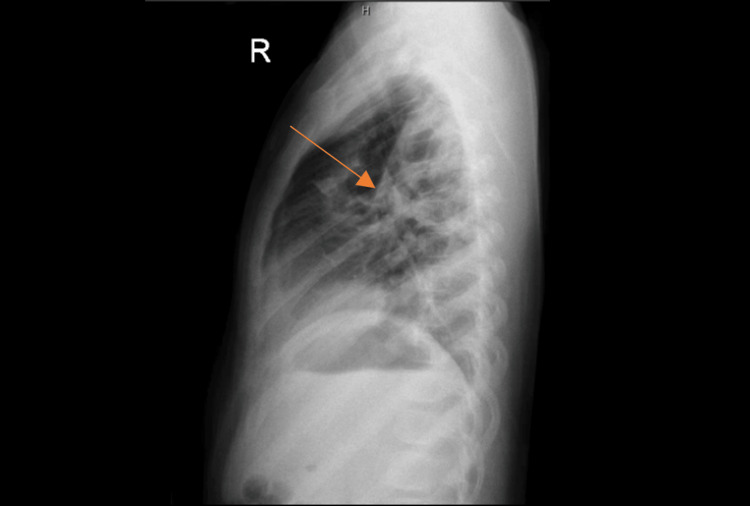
Chest X-ray. Cavitary lesions with air-fluid levels in the upper lung. The arrow pointing to the air-fluid level.
